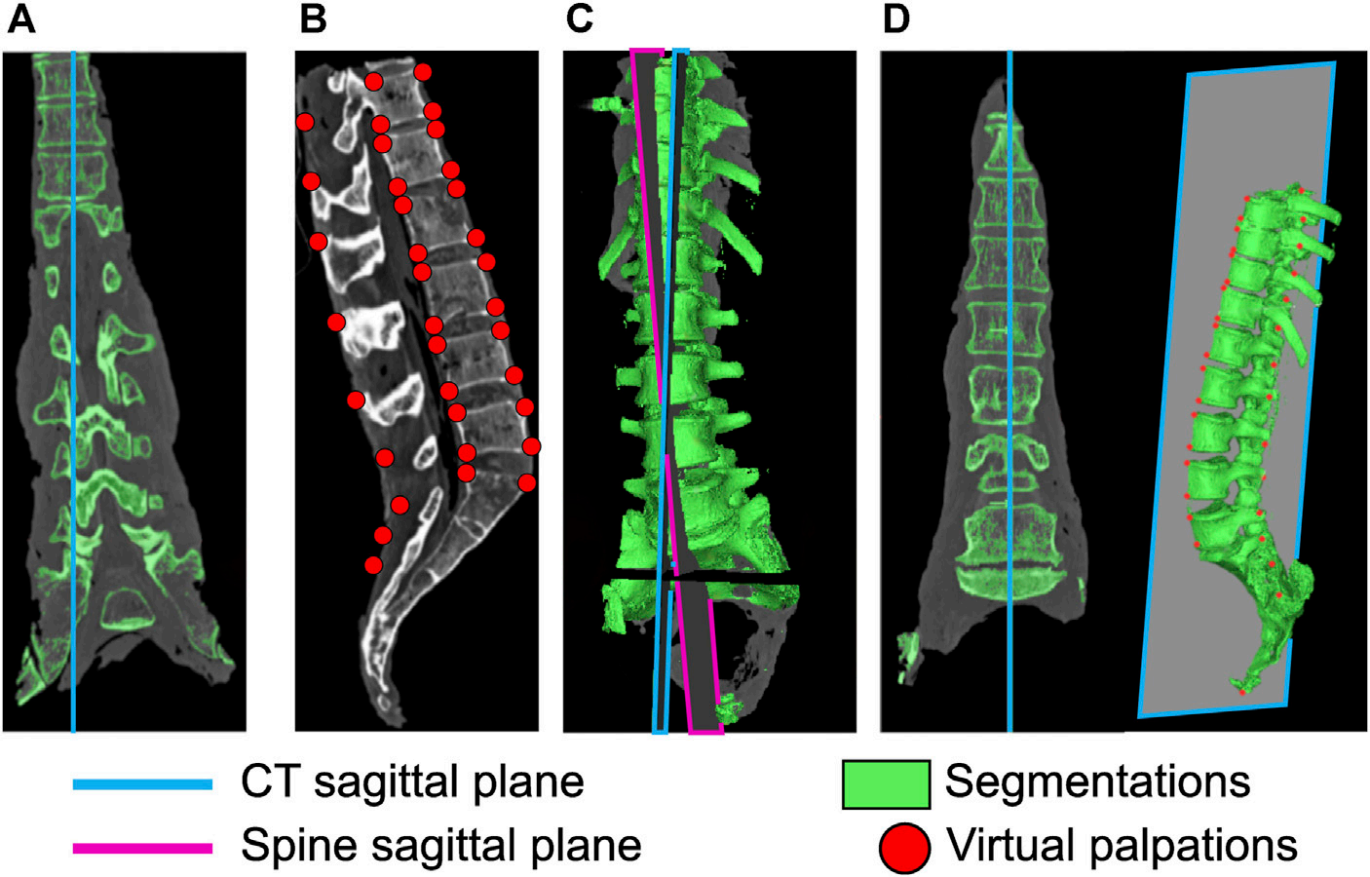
Realignment of the CT sagittal plane. (A) original sagittal plane of the CT data, (B) virtual palpation of landmarks defining the spine sagittal plane, (C) definition of the spine sagittal plane and the sagittal plane of the CT data, (D) CT data after realigning the CT sagittal plane to the spine sagittal plane.
